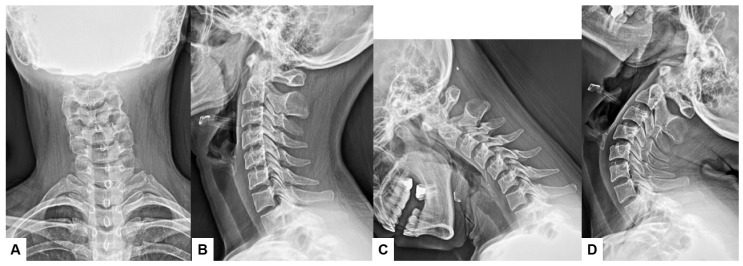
Thirty-four-year-old F, cervical spondylosis, (A) anteroposterior cervical conventional X-ray, (B) lateral conventional X-ray, (C) lateral flexion X-ray, (D) lateral extension X-ray. Cervical motion is preserved, and there is no instability.
